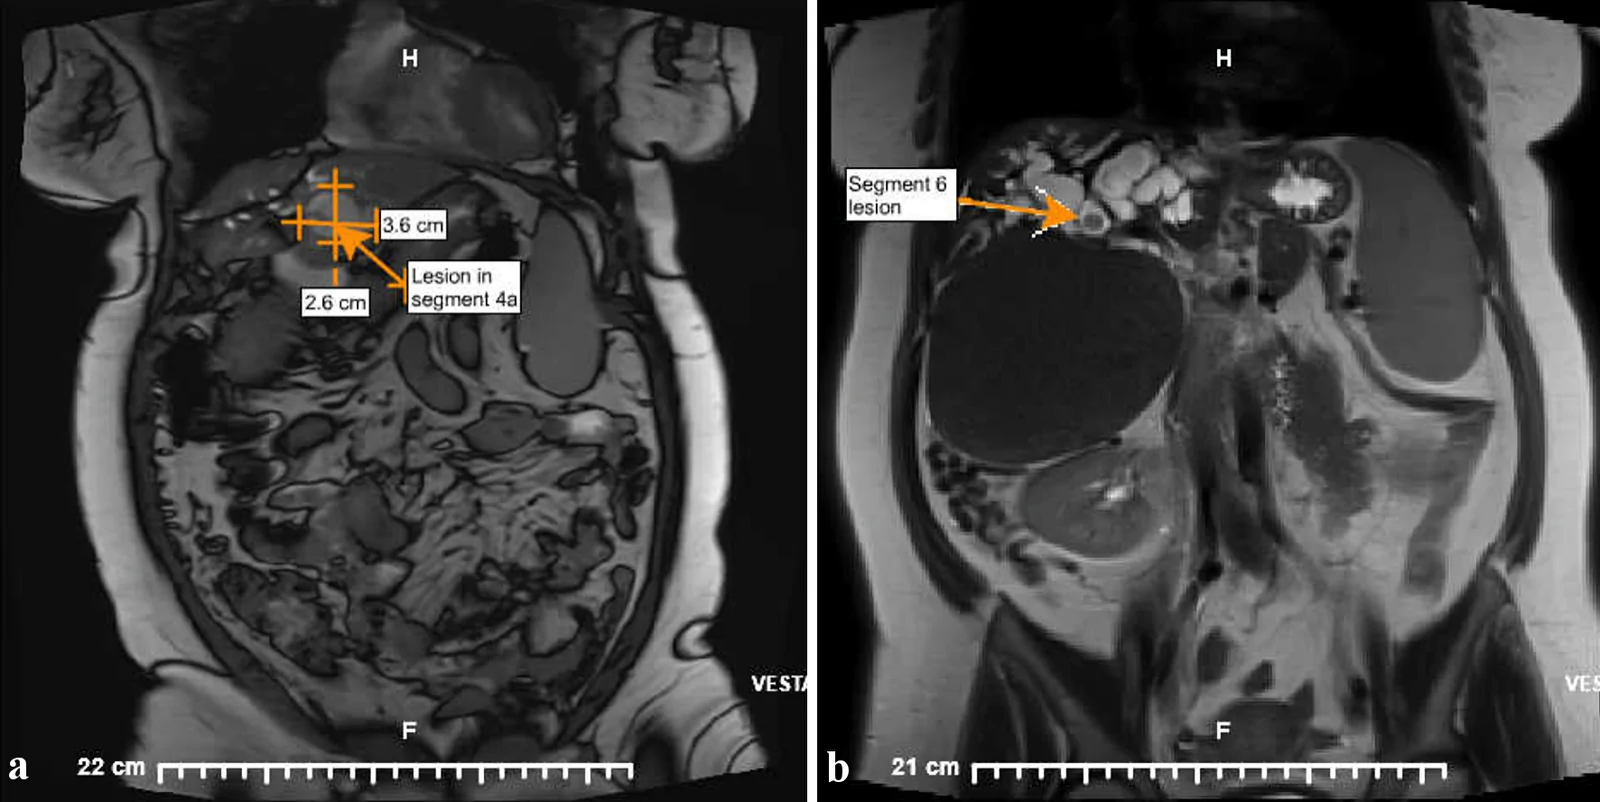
Magnetic resonance imaging with gadolinium of abdomen–pelvis, showing (a) an arterially enhancing lesion measuring 3.6 × 2.6 cm in segment 4a and (b) a second exophytic lesion arising from segment 6 which demonstrated different signal characteristics.
